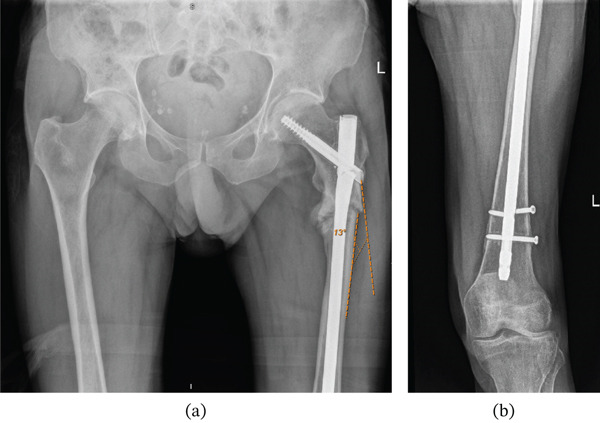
(a) 8‐month postoperative anteroposterior radiographic imaging after initial procedure showing nonunion at the left femur fracture site and a broken proximal nail. Noted varus deformity (measured 13°) and (b) 8‐month postoperative anteroposterior radiographic imaging after initial procedure showing a broken distal locking screw.
